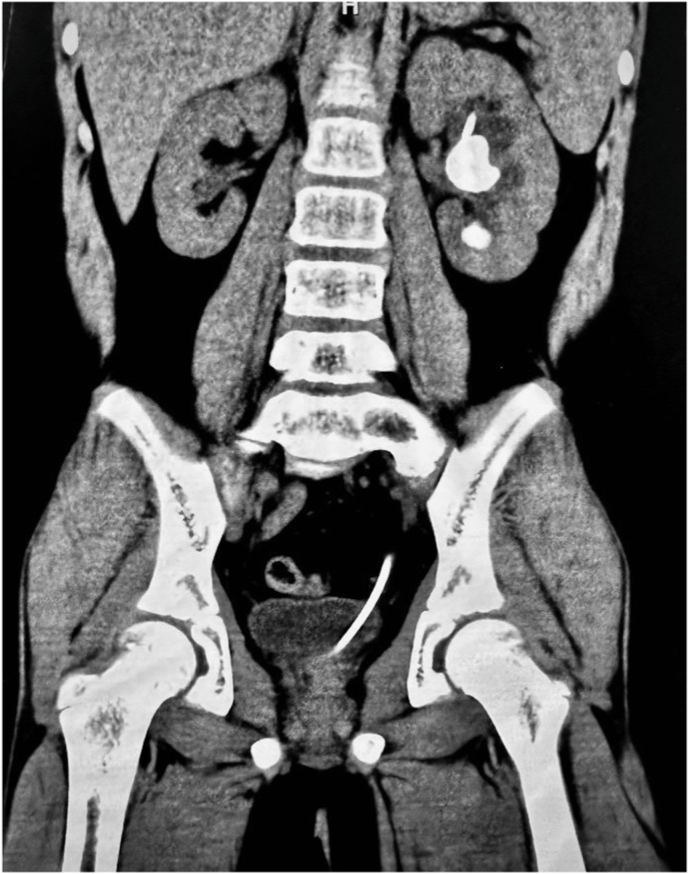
Preoperative non-contrast computed tomography image showing a 3.0 cm renal pelvic stone and a 0.7 cm lower pole stone in the left kidney.
